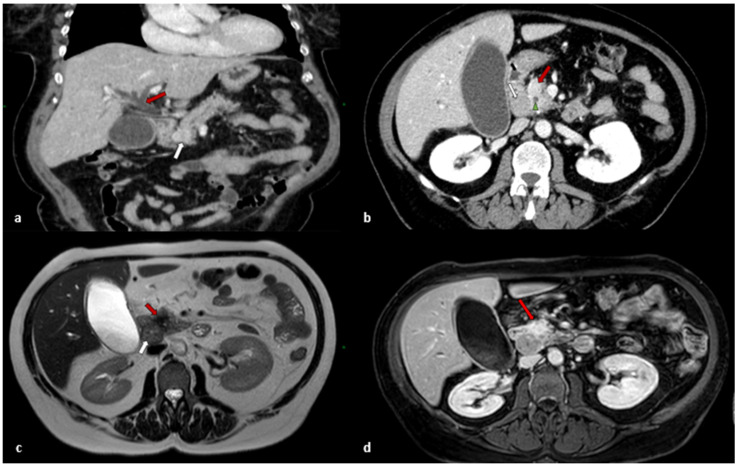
(a) Post-contrastographic CT scan coronal view shows inhomogeneous enhanced expansive solid mass with irregular edges localized at pancreatic cephalic portion (white arrow). The mass exerts compressive effect on the duodenum and choledochus, with evident dilatation of extra- and intra-hepatic biliary ducts upstream (red arrow); (b) Contrast-enhanced CT scan axial view shows inhomogeneous enhanced mass with irregular edges localized at cephalic portion of pancreas (red arrow). Made evident are the dilated choledochus in the context of the mass (green arrowhead) and the presence of enlarged, globose, and inhomogeneous lymph node (white arrow) localized in peri-duodenal region, which is suspicious for metastasis; (c) MRI axial T2-weighted image shows the lesion as hypointense area with badly defined spiked edges lesions (red arrow). It also shows the enlarged, globose metastatic lymph node, which appears slightly and inhomogeneously hypointense (white arrow); (d) MRI gadolinium-enhanced T1-weighted axial image with fat suppression shows intense signal enhancement of the lesion (red arrow).
